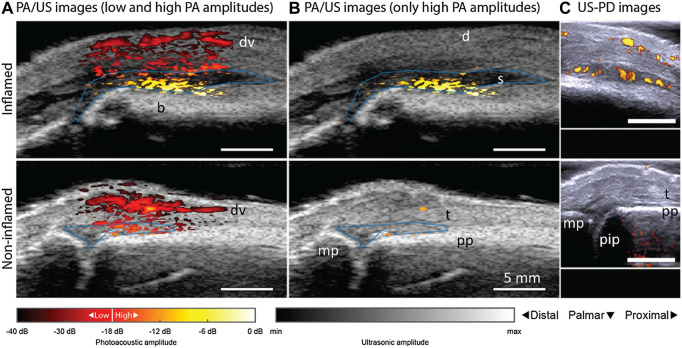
PAT/US and US/PD images of an inflamed contra-lateral (bottom row) and non-inflamed (upper row) joint from a patient with rheumatoid arthritis. PAT/US images in (A) show a difference in color between inflamed and non-inflamed corresponding to an increase in amplitude levels. If low PAT amplitudes are discarded in (B), only features in the inflamed joint are seen. Figure (C) shows the corresponding US-PD images. Blue lines in PAT/US images indicate ROIs used for PAT feature quantification in synovial space. The 0 dB level is the maximum PAT amplitude produced by an inflamed joint. d, dermis; dv, dorsal vein; pp, proximal phalanx; pip, proximally located interphalangeal joint; mp, midline phalanxes; s, synovium; t, extensor tendon; PAT, photoacoustic tomography; US, ultrasound; PD, powered Doppler. Reprinted with permission from [van den Berg P. J., Daoudi K., Bernelot Moens H. J., and Steenbergen W. (2017). Feasibility of photoacoustic/ultrasound imaging of synovitis in finger joints using a point-of-care system. Photoacoustics. 8,8–14. doi: 10.1016/j.pacs.2017.08.002.
